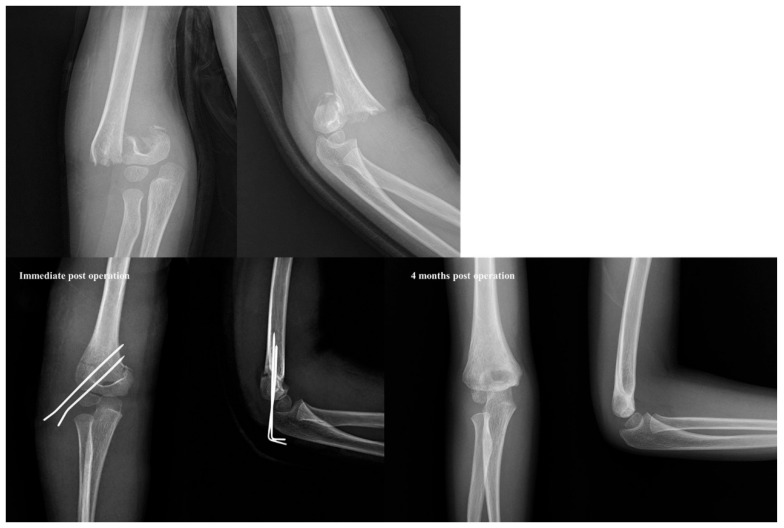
Example of a low transverse fracture type in a 6-year-old boy with a Gartland type III supracondylar fracture. Despite being a Gartland type III fracture, the internal rotational stress test was negative, even with only two lateral pins fixation. Furthermore, there was no loss of reduction in the radiographs taken 4 months post-operation.
